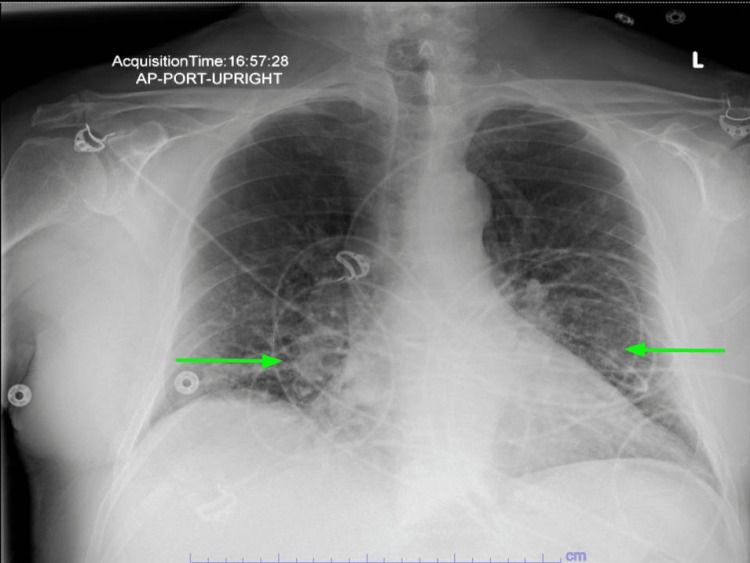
Chest x-ray showed streaky bibasilar airspace disease (green arrows).
